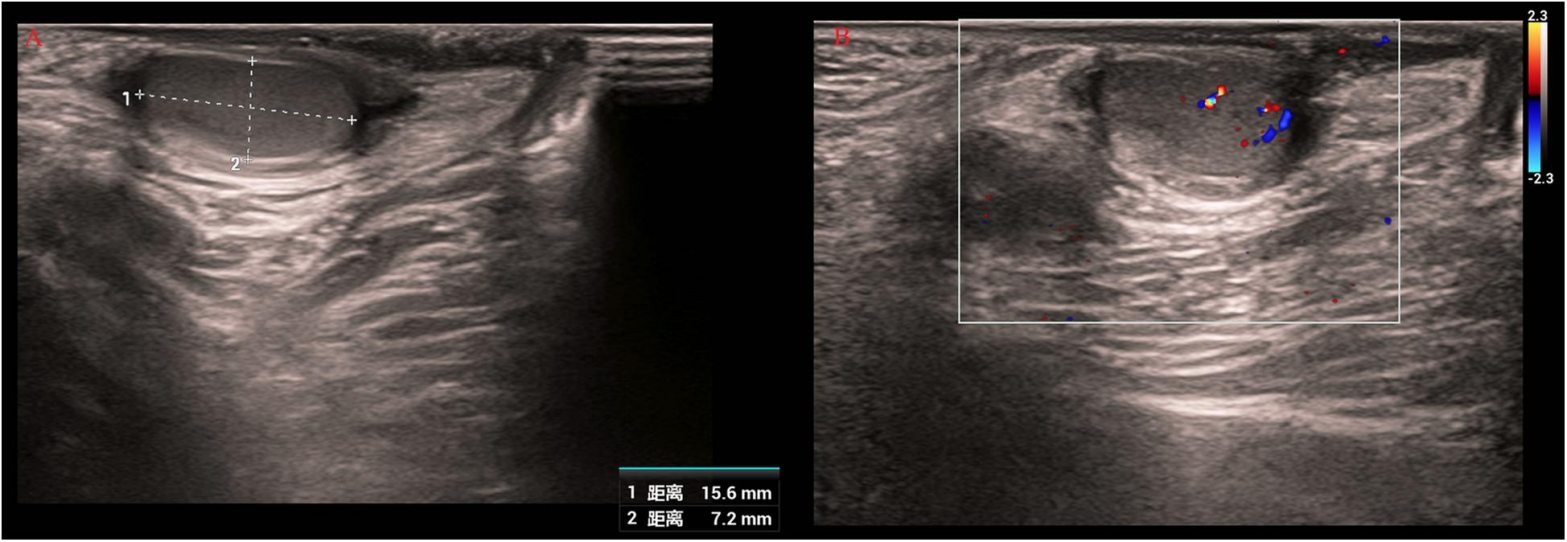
Postoperative scrotal ultrasound of the left testis. (A) Gray-scale image showing preserved parenchyma with normal echogenicity. (B) Color Doppler image demonstrating normal vascular flow without evidence of residual tumor or recurrence.
